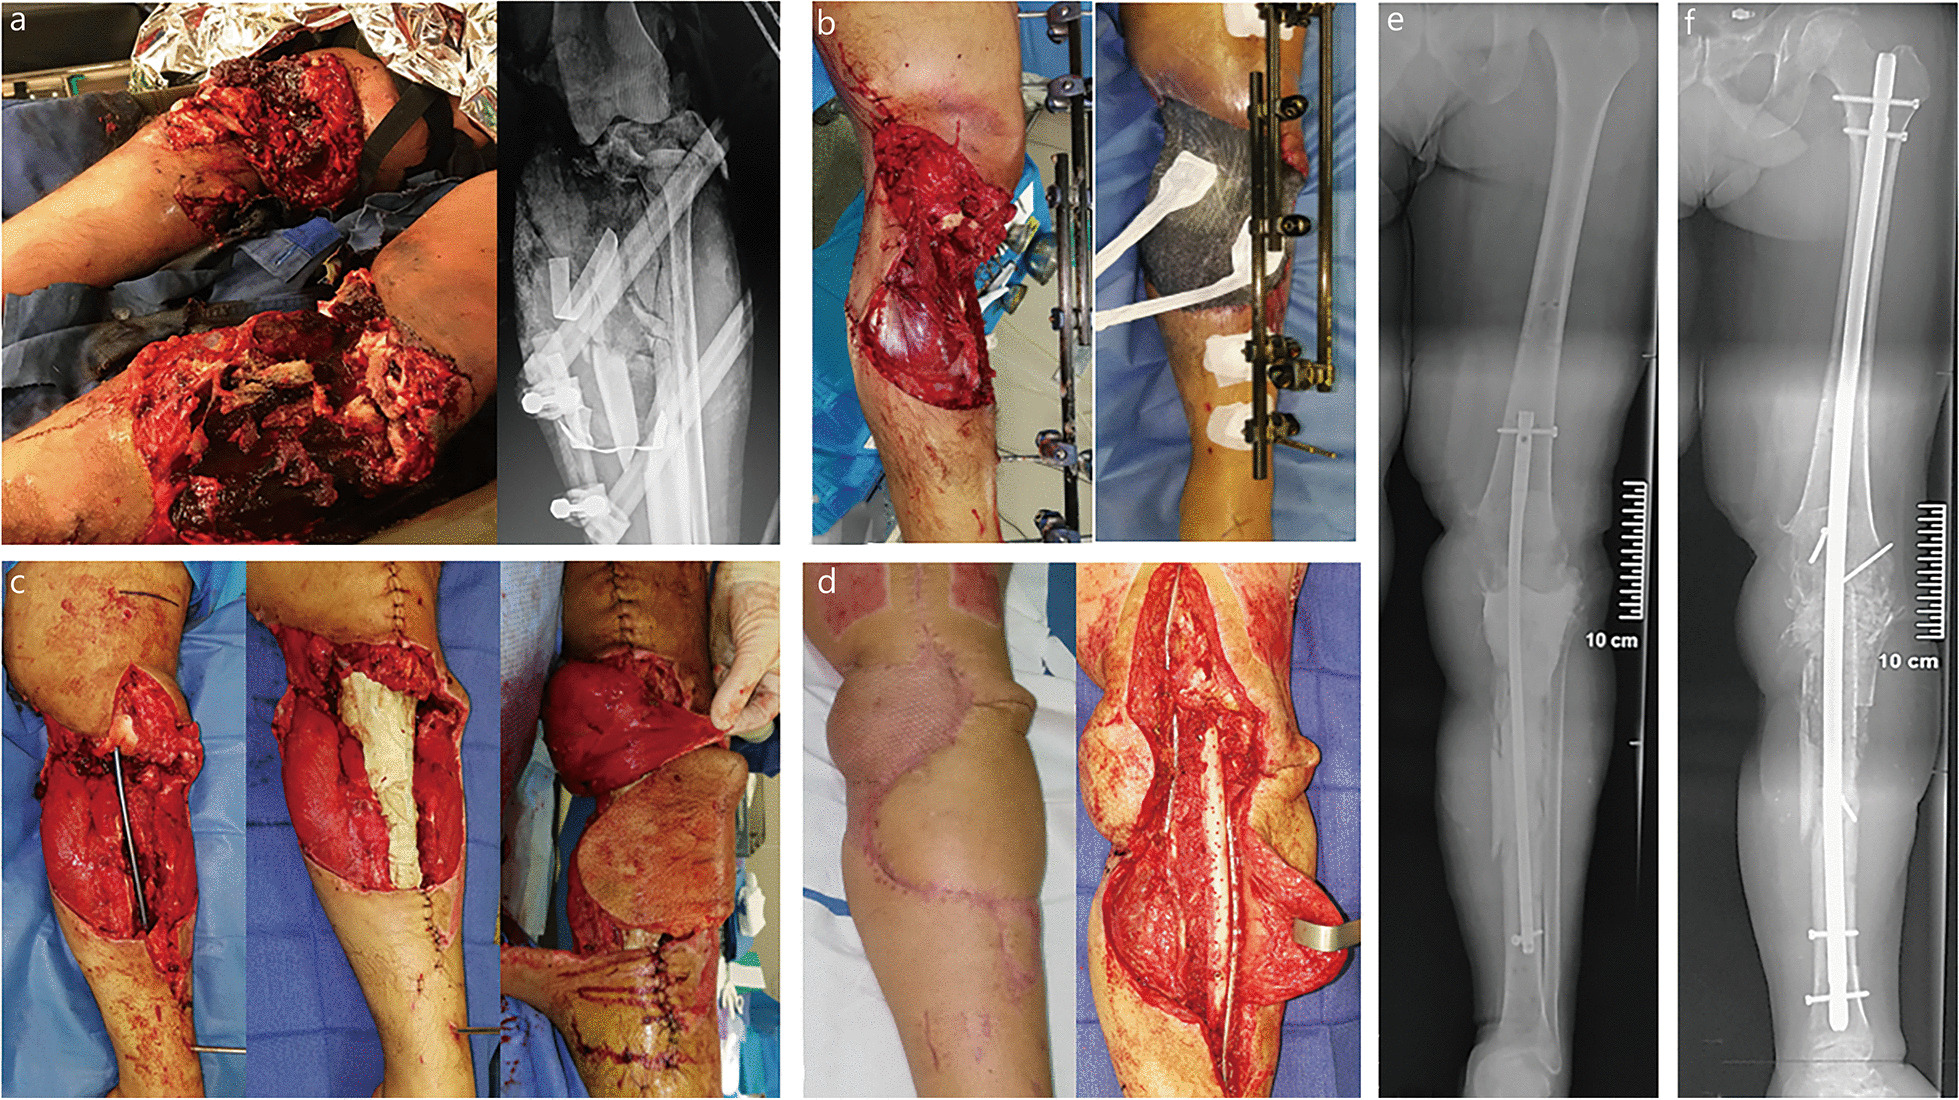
Reconstruction of a massive multi-tissue defect of the left knee and proximal tibia (SOFCOT type 4) following a helicopter crash. a Clinical and radiological view at admission. b Primary management according to DCO principles. c Implantation of a 22 cm-long reinforced spacer and flap coverage with combination of medial gastrocnemius muscle and proximal sural flaps at stage 1 (a free flap transfer was precluded by multiple venous thromboses). d Operative views during bone grafting associating cancellous bone grafts and a multiperforated non-vascularized fibular strut. e X-rays showing the reinforced spacer after stage 1. f Radiological view after stage 2: a knee arthrodesis was performed using a femorotibial nail. Progressive weight bearing was initiated at month-3. Bone union was achieved after 6 months without any complication. DCO damage control orthopedics, SOFCOT *Société Française de Chirurgie Orthopédique et Traumatologique*
